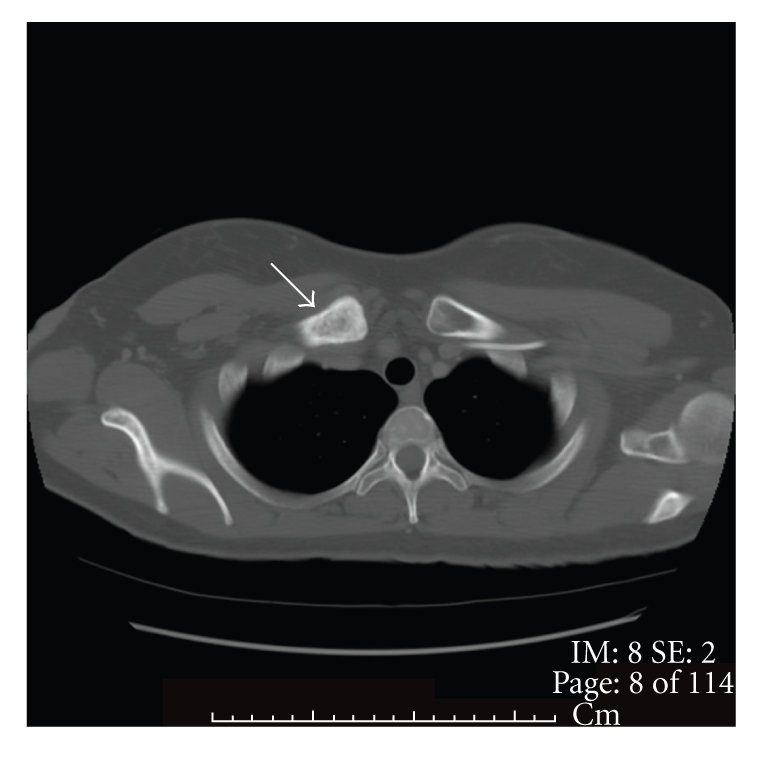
CT scan with contrast through the clavicle. There is increased sclerosis of the medial right clavicular head (white arrow) with a moth-eaten appearance of the cortex and medullary space. The differential diagnosis is metastasis or chronic osteomyelitis. Biopsy subsequently showed metastatic pancreatic cancer.
